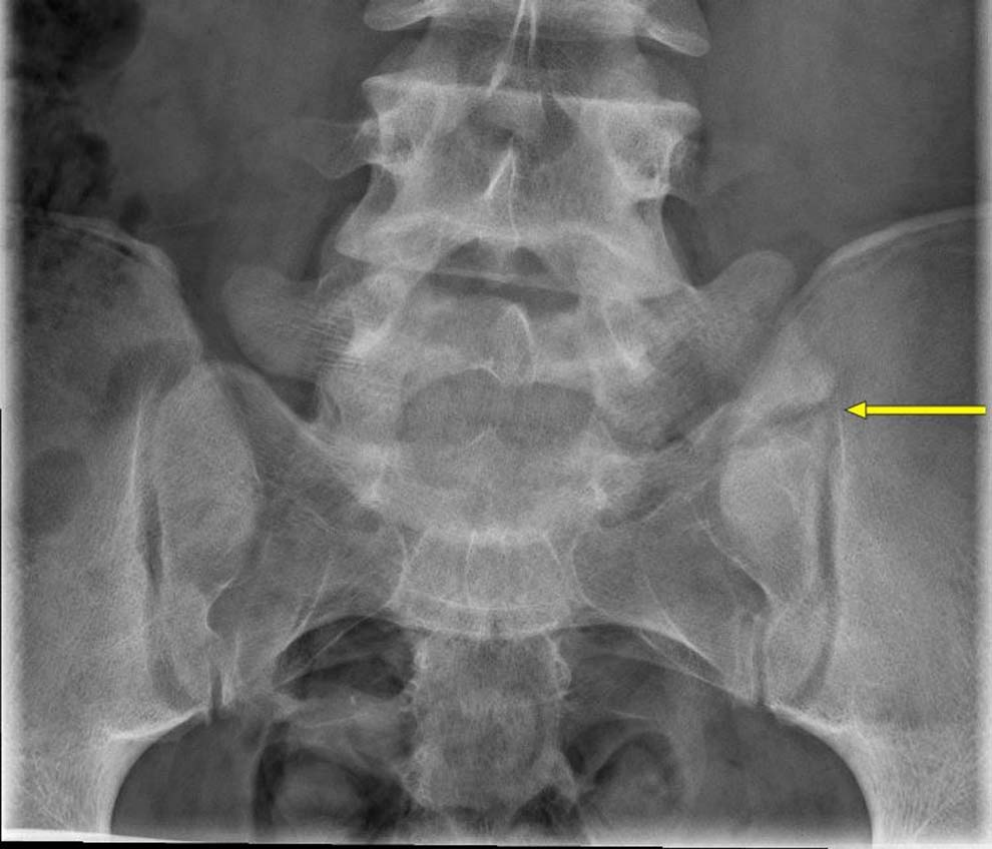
Anteroposterior lower lumbar spine preoperative radiograph demonstrates prominent L5 transverse processes bilaterally (left greater than right). The left transverse process appears to articulate with the sacrum (arrow demonstrates articulation).
